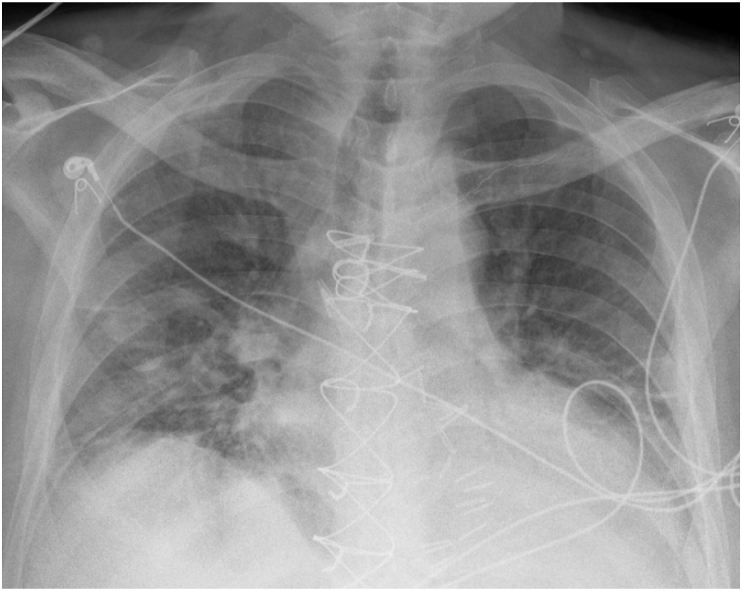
Chest radiograph of Case 1 on hospital day 8 post-extubation which shows improvement in multifocal airspace opacities.
